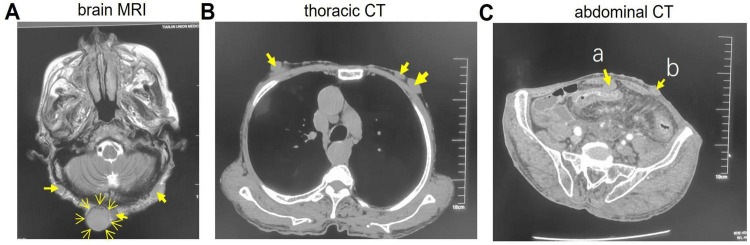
Imaging studies data. (A) Brain magnetic resonance imaging (MRI). (B) Thoracic CT. (C) Abdominal CT. The arrows indicate nodules. a, Omentum nodule; b, Abdominal nodule.
